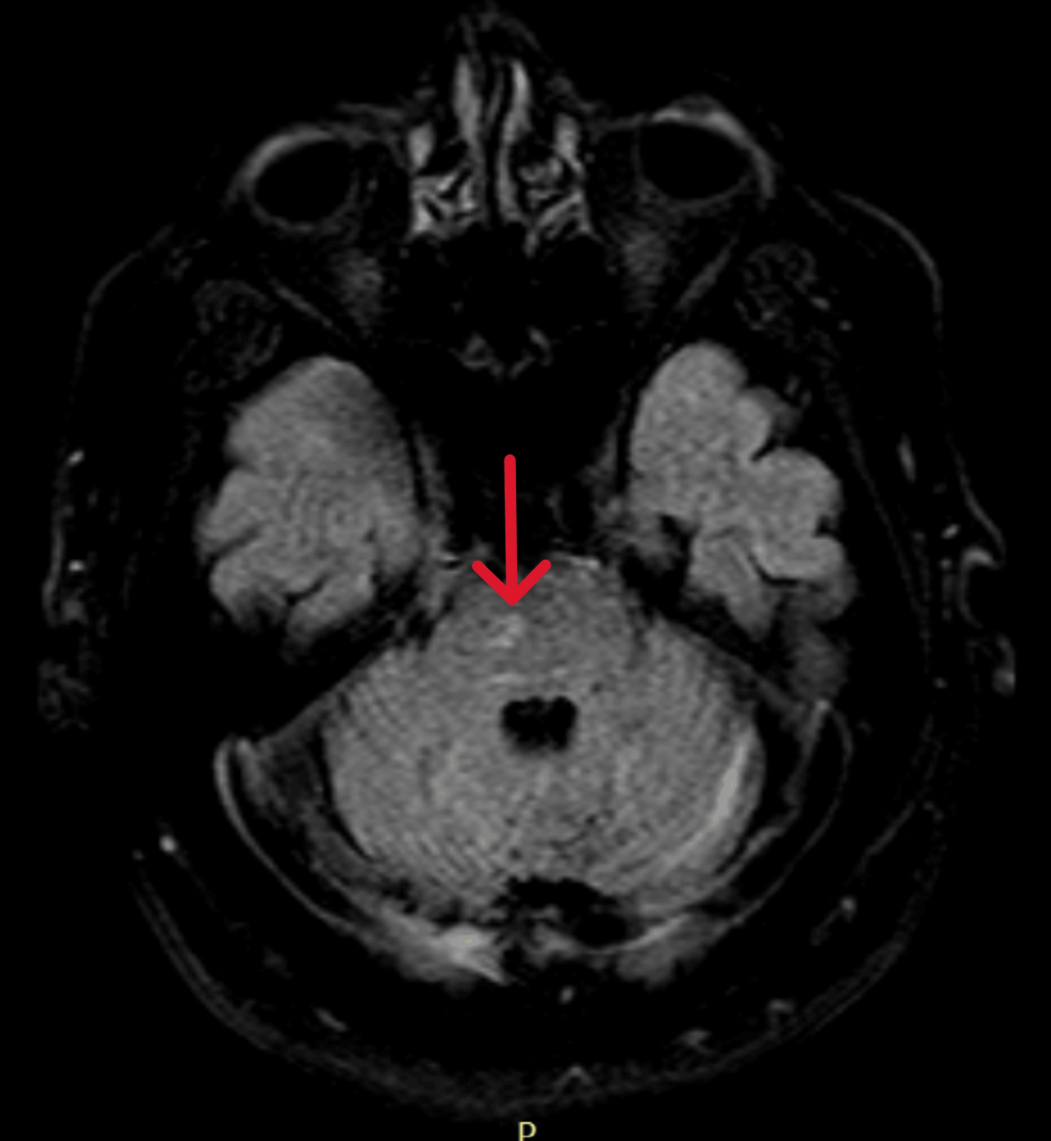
Axial FLAIR MRI of the brain showing a small right pontine lacunar infarct and cortical atrophy (arrow) FLAIR: fluid-attenuated inversion recovery
